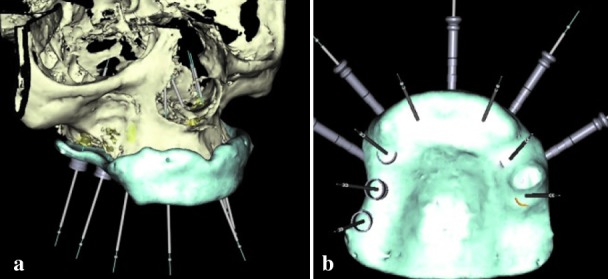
a: Implants originally planned on modified radiographic template can now be visualized relative to the contours of the original radio-graphic template, b: Anchors pins added to implant planning
